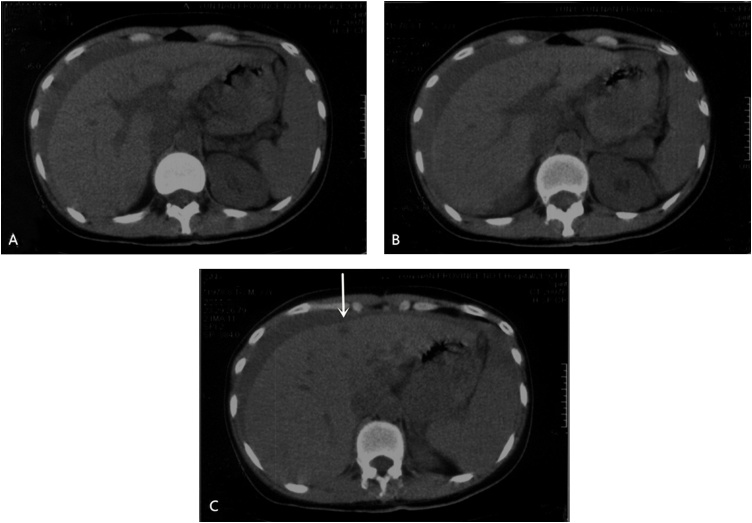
Immediate abdominal computed tomography revealing ascitic fluid in the peritoneal cavity, lymph node enlargement of hepatic portal (A, B) and a 19 × 13 mm^2^ metastatic lesion in the left medial segment of the liver (arrow) in August 2011.
